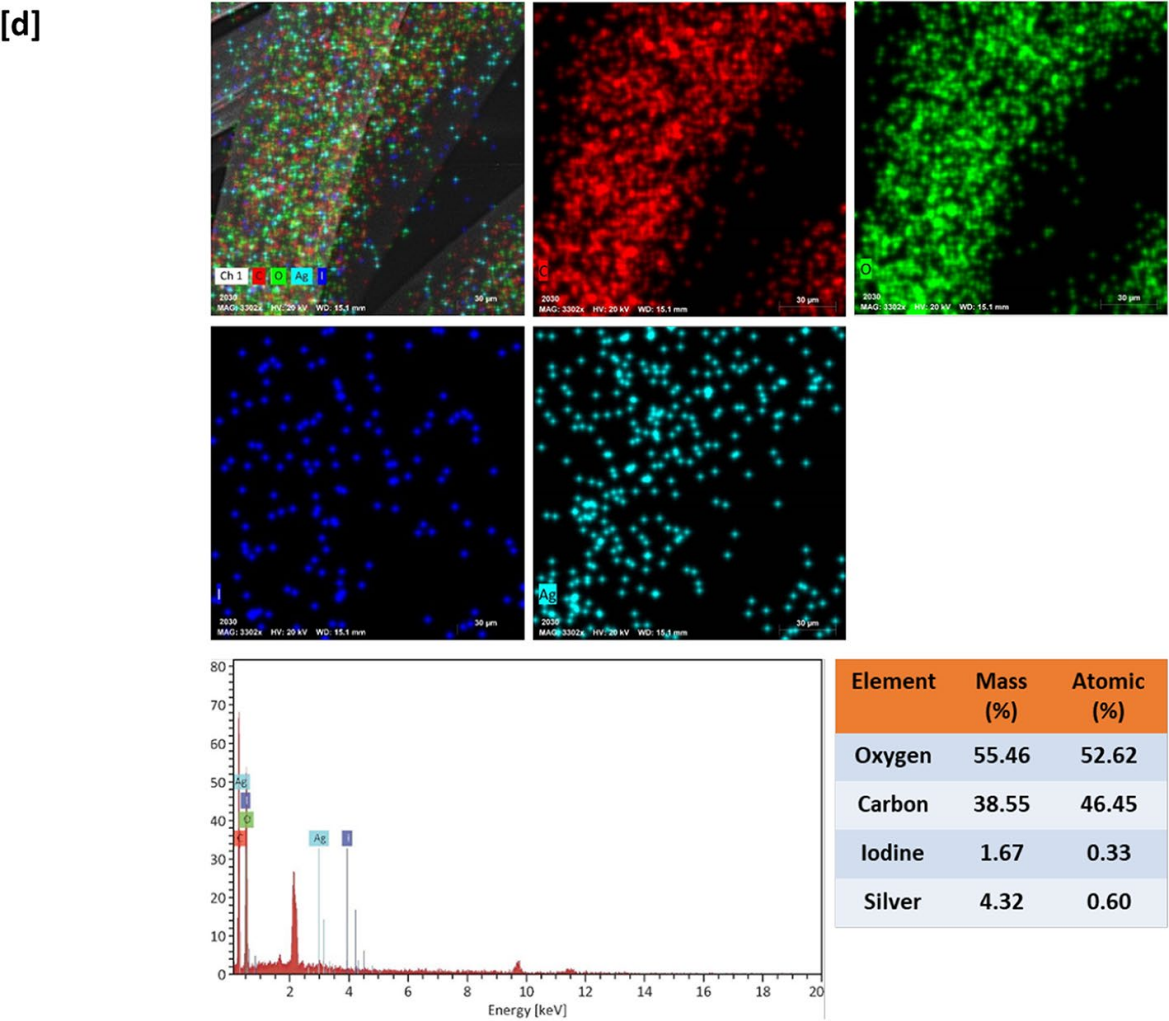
Mapping and energy dispersive X-ray analysis for the bamboo fabric samples; (a) bamboo blank, (b) AgI@bamboo, (c) Ag NPs@bamboo, and (d) Ag NPs@AgI@bamboo
